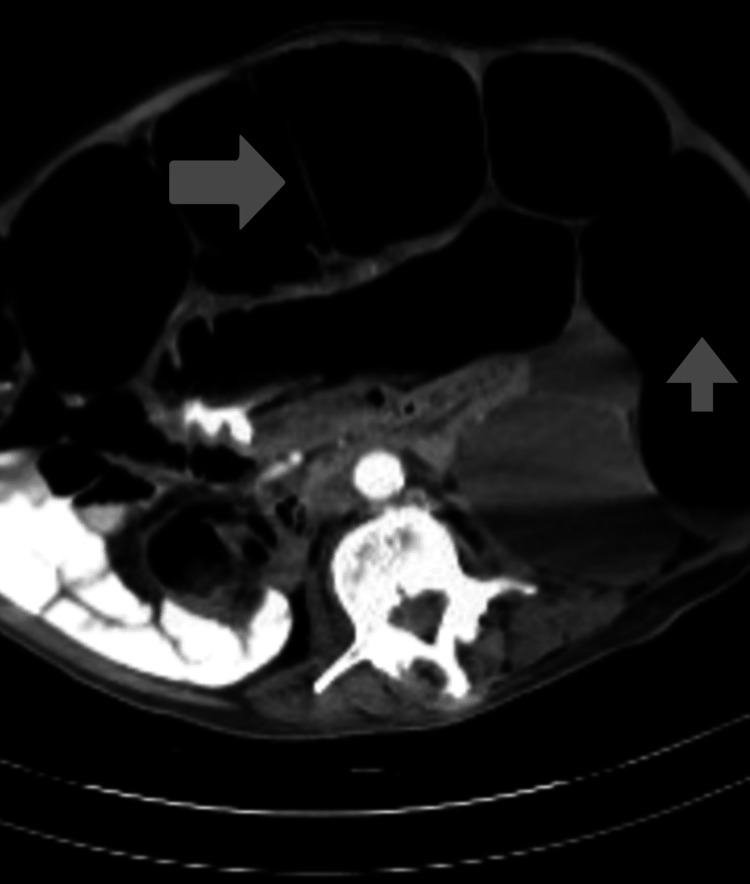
CT scan transverse view of the abdomen. CT scan transverse view showing marked gaseous distention of the colon, which may represent a megacolon. No obvious obstructing lesion noted.
